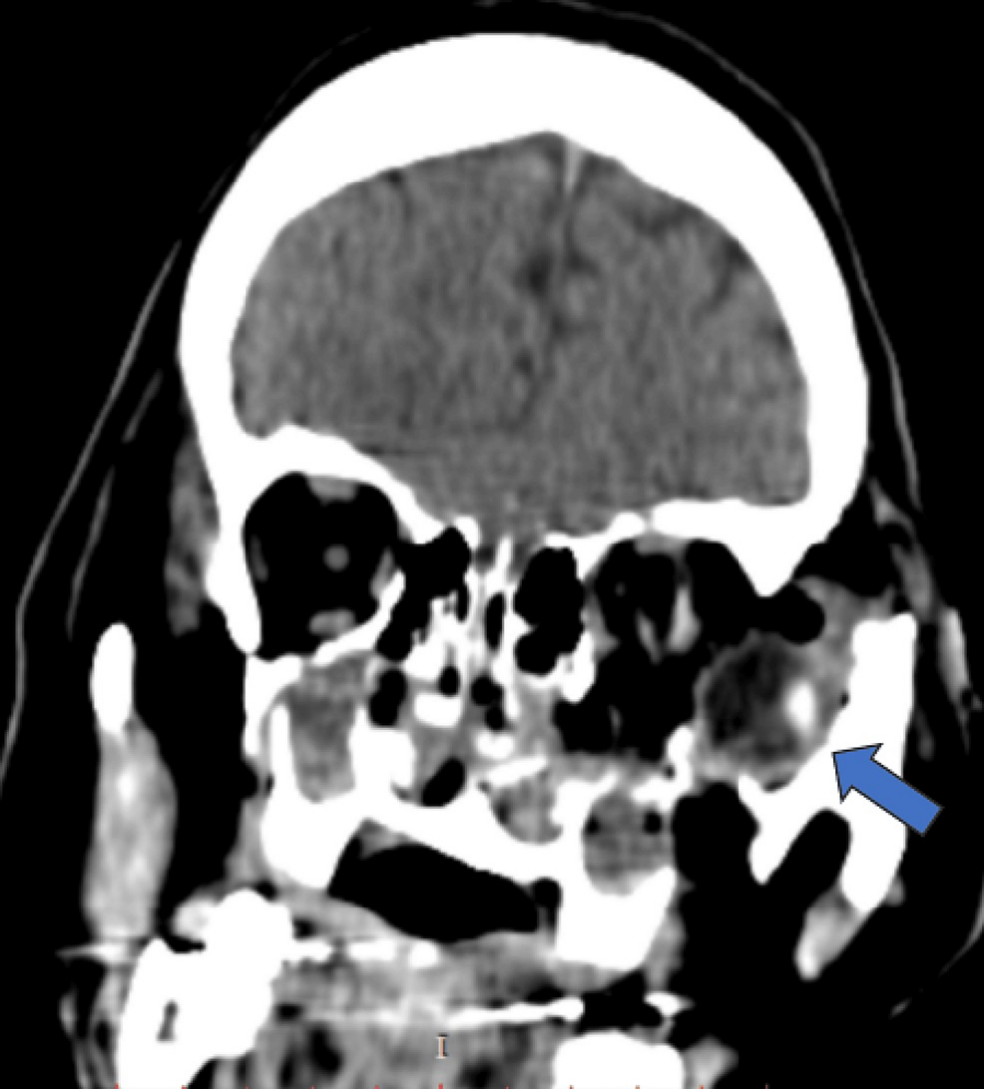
CT scan (coronal view) showing left globe luxation inferotemporally (blue arrow) with fracture over the floor and the lateral wall of the left orbit.
